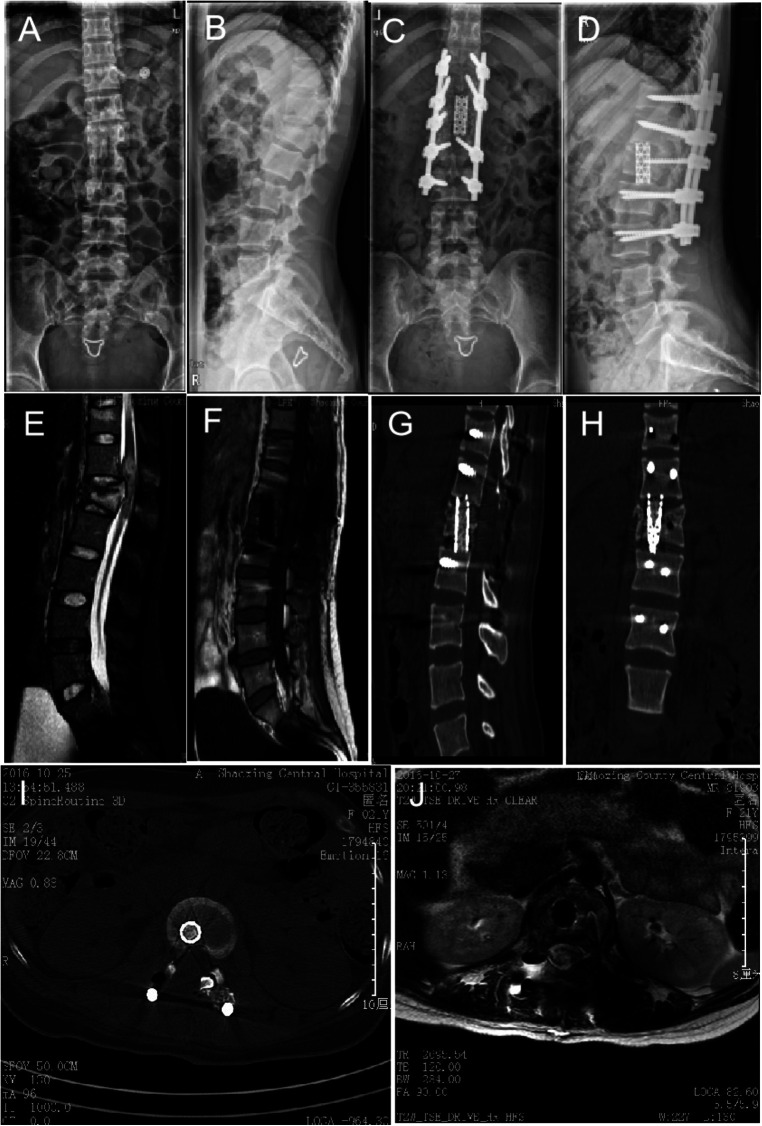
Typical preoperative, postoperative, and follow-up images of severe thoracolumbar burst fractures with posterior midline spinal approach for spinal canal limited decompression and 13-mm titanium mesh implantation (case #2). (A,B) True lateral digital x-rays of the patient's thoracolumbar spine before the operation showing the L1 fracture. (C,D) True lateral digital x-rays of the thoracolumbar vertebrae after the operation showing good fixation and recovery of the height of the anterior edge of the L1 vertebra. (E,F) Preoperative magnetic resonance imaging (MRI) showing L1 burst fracture, spinal cord compression, and obvious spinal canal occupancy. (G–J) Postoperative MRI indicates no spinal cord compression and no spinal canal occupancy. (I) Postoperative computed tomography (CT) indicates a good position of the titanium mesh, screws, and titanium rods.
